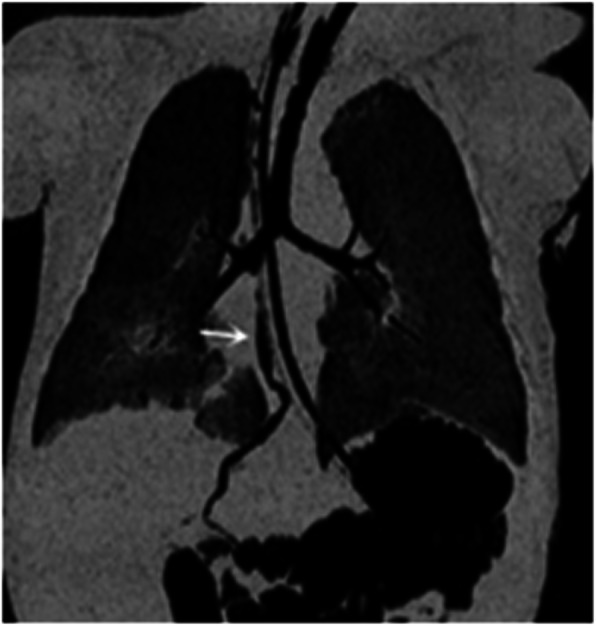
Three-dimensional chest computed tomogram showing the fistula originating from the right bronchus, travelling along the esophagus, and passing through the diaphragm and into intrahepatic biliary tract at the site of the esophageal hiatus
